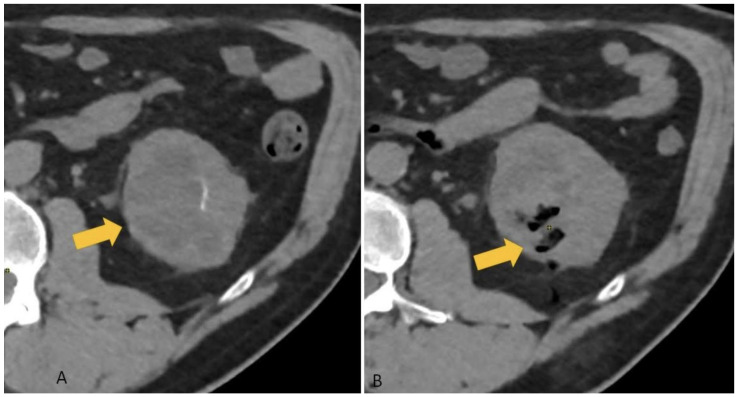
(A) Suspected renal mass (yellow arrow)—target to CT-guided percutaneous biopsy, (B) post-biopsy non-contrast CT scan, showing the dissemination of the gelatin foam paste along the site of the biopsy to the renal capsule.
